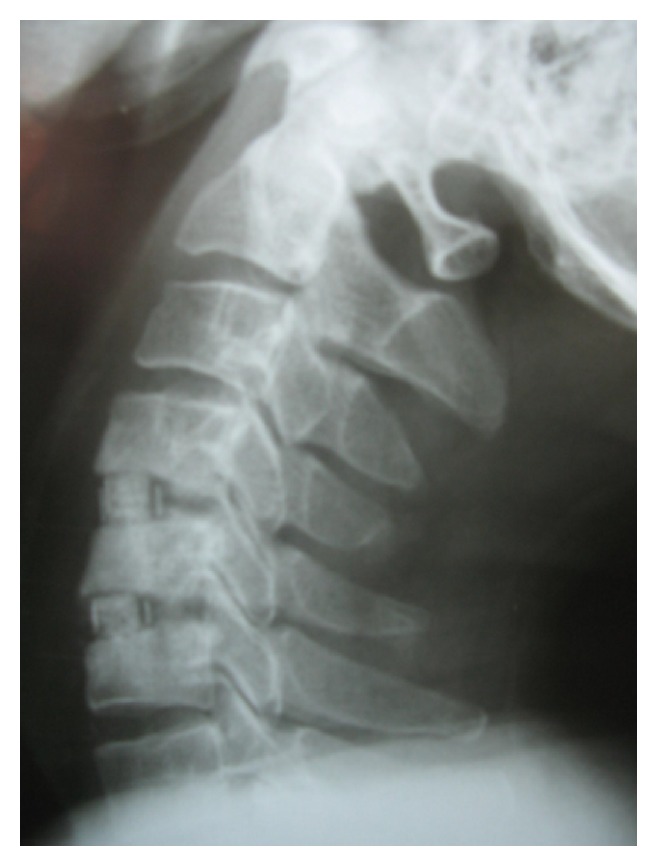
Postoperative X-ray of 2-level ACDF.
